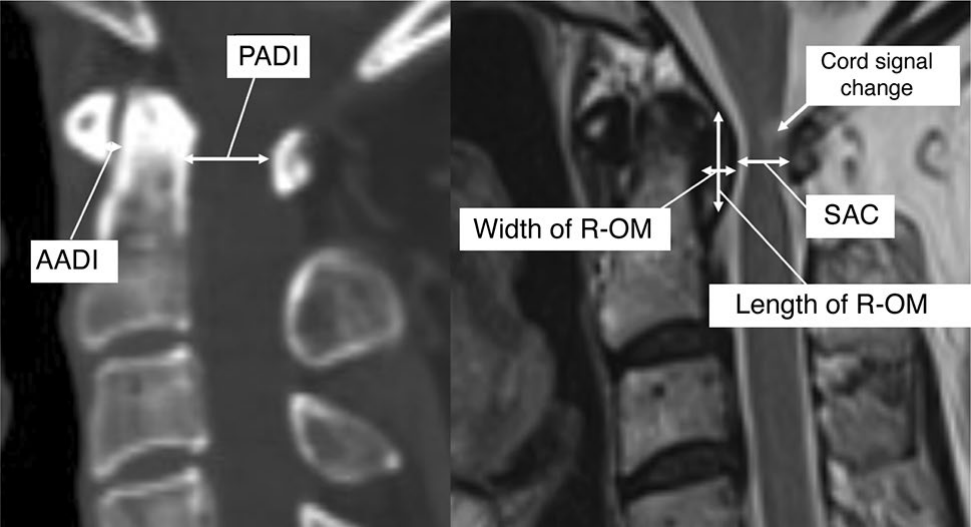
Radiographic parameters were measured on the computed tomography and T2-weighted sagittal cervical spine magnetic resonance images. *AADI* anterior atlantodental interval, *PADI* posterior atlantodental interval, *R-OM* Retro-odontoid mass, *SAC* space available for the spinal cord.
